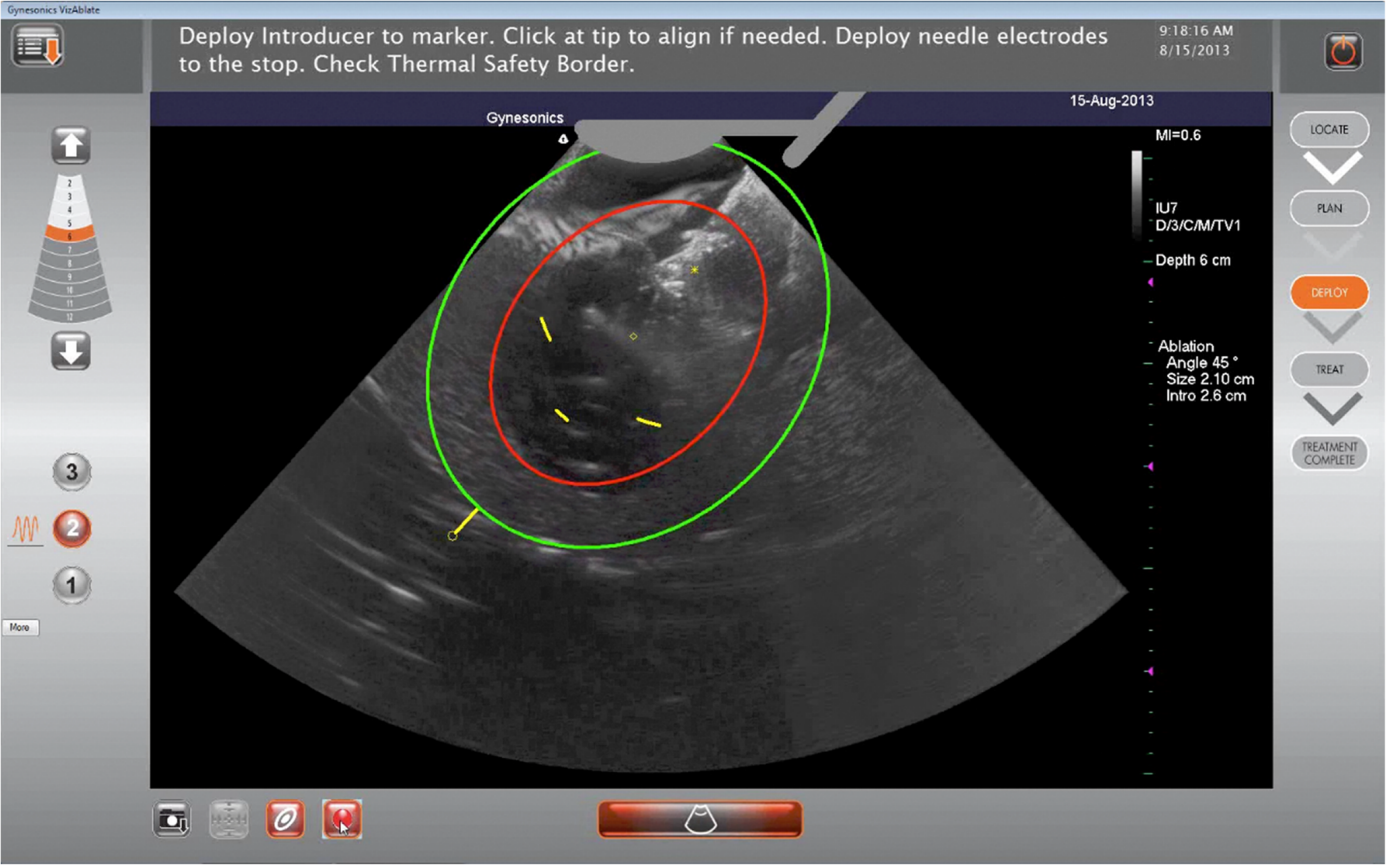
Intrauterine sonogram of a submucosal fibroid with the VizAblate ablation guides visible (Ablation Zone in *red* and Thermal Safety Border in *green*). The Ablation Zone is a two-dimensional representation of the average region of tissue ablation for a selected treatment size. Tissue outside the Thermal Safety Border is at a safe distance from the Ablation Zone and will be preserved. The serosa is visible as an echogenic border around the uterus
